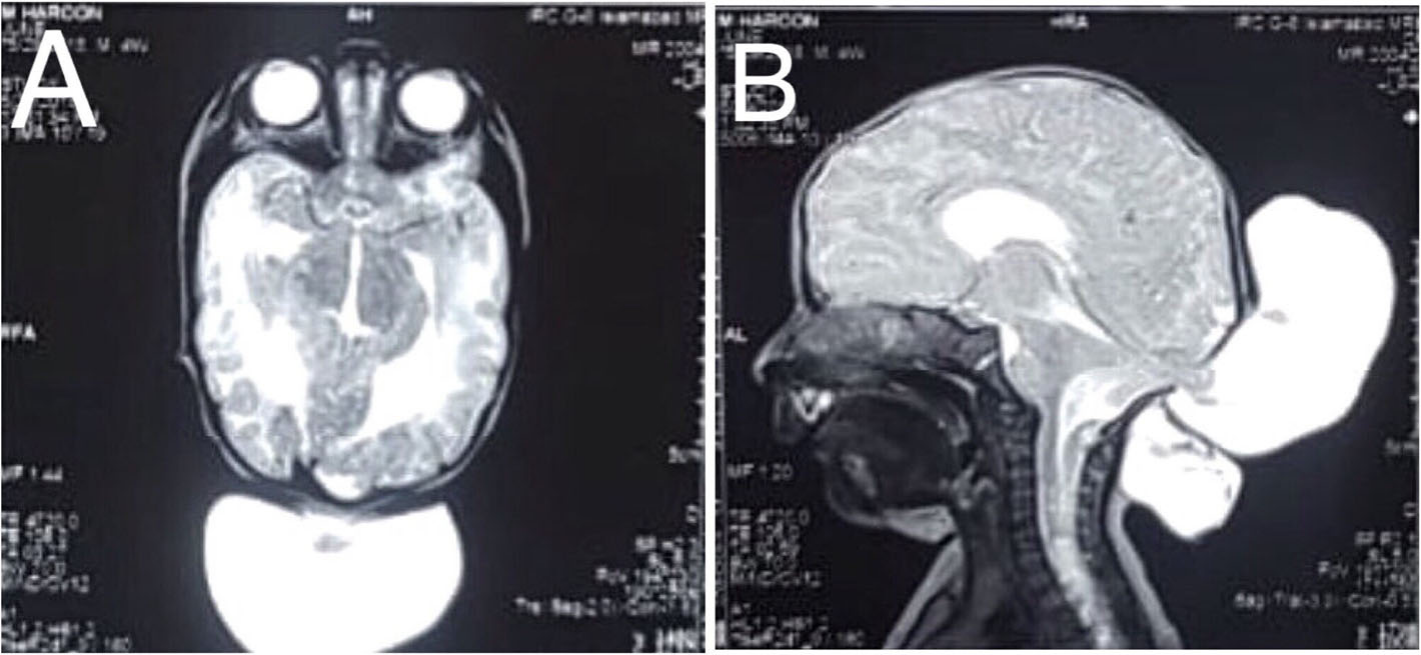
MRI Brain a & b showing Occipito-cervical meningoencephaloceles
